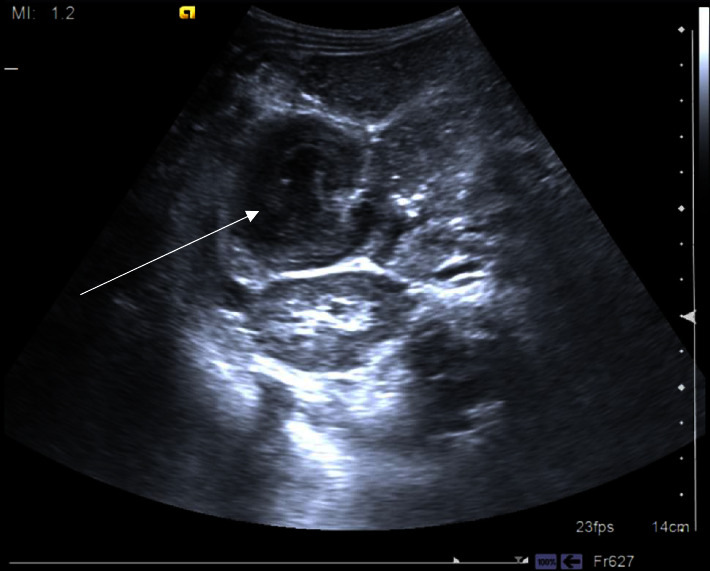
Abdominal ultrasound. Ultrasound showing target sign (arrow), which is suggestive of intussusception.
